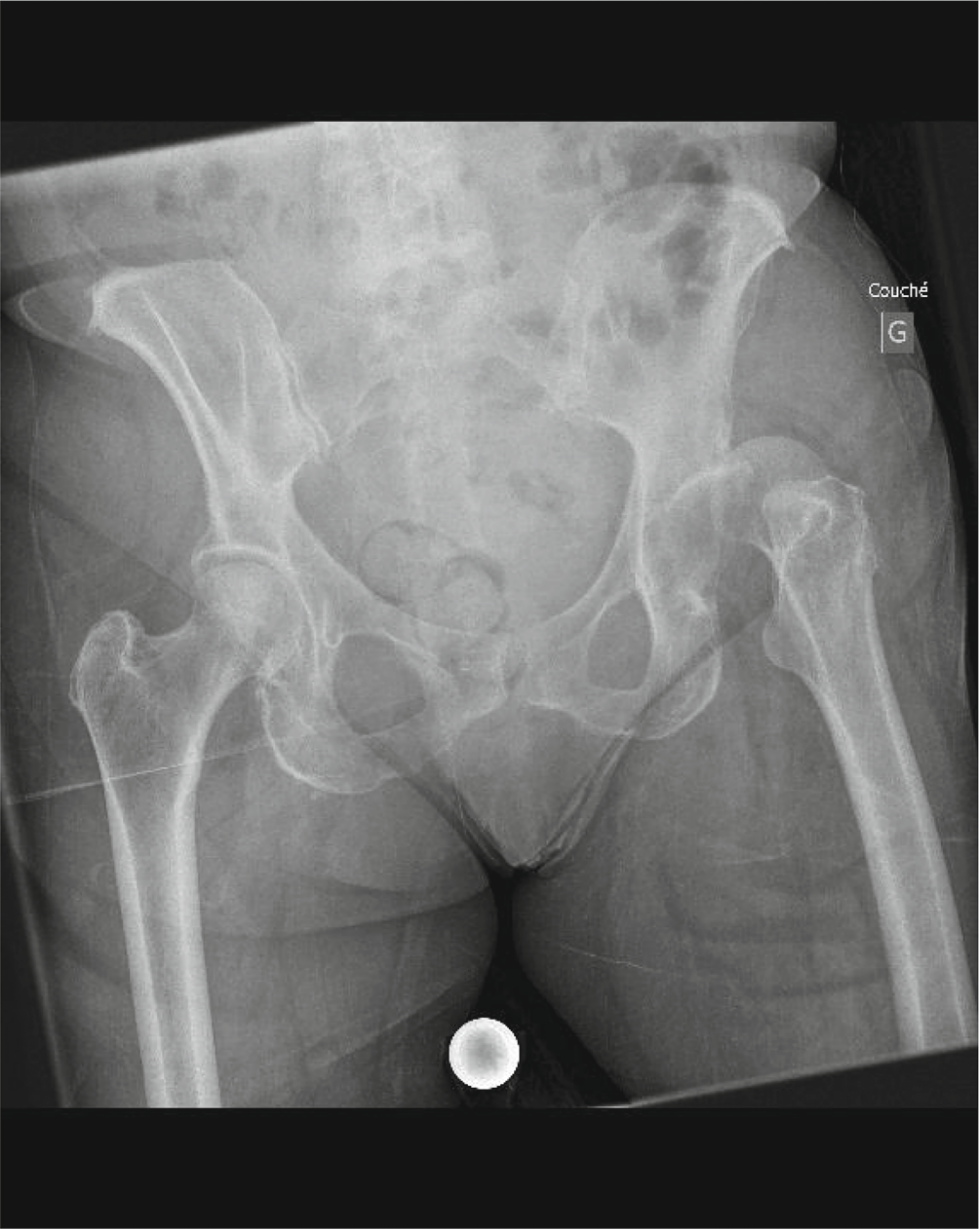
Anteroposterior radiograph of the pelvis showing anterior superior dislocation of the left hip.
